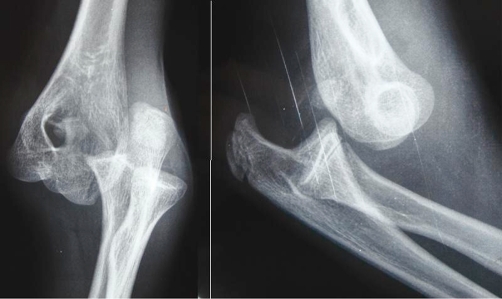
Radiographs showing a posterolateral dislocation of the elbow. (A) Anteroposterior view. (B) Lateral view.
